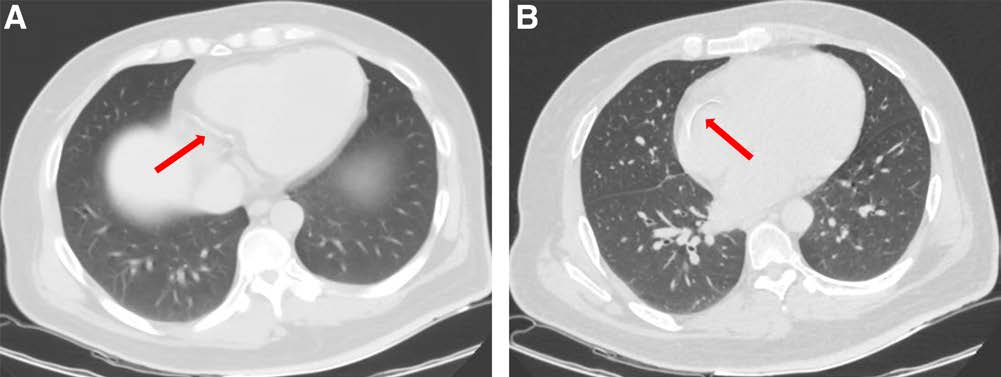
(A and B) Preoperative chest computed tomography (CT) scan, The red arrow points to coronary arteriosclerosis.
